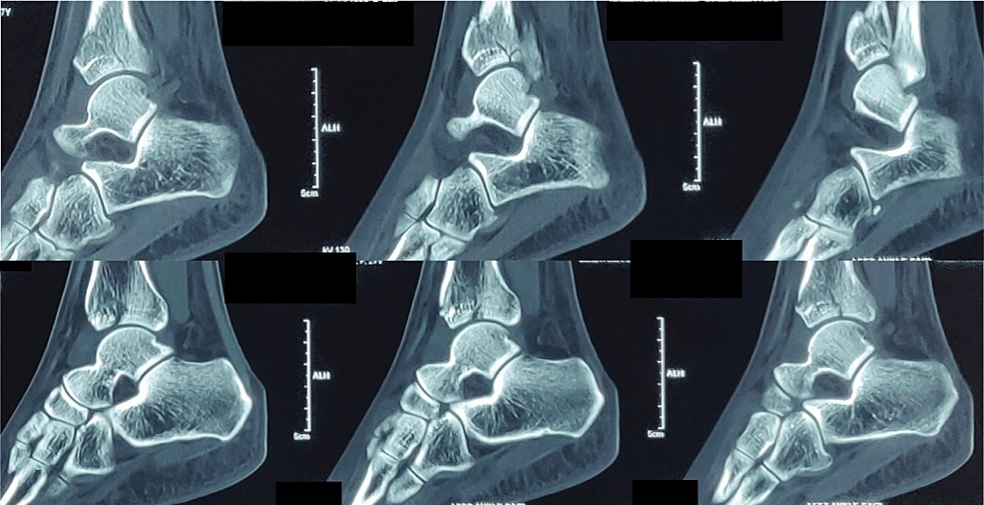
Saggital sections of CT scan of affected ankle
